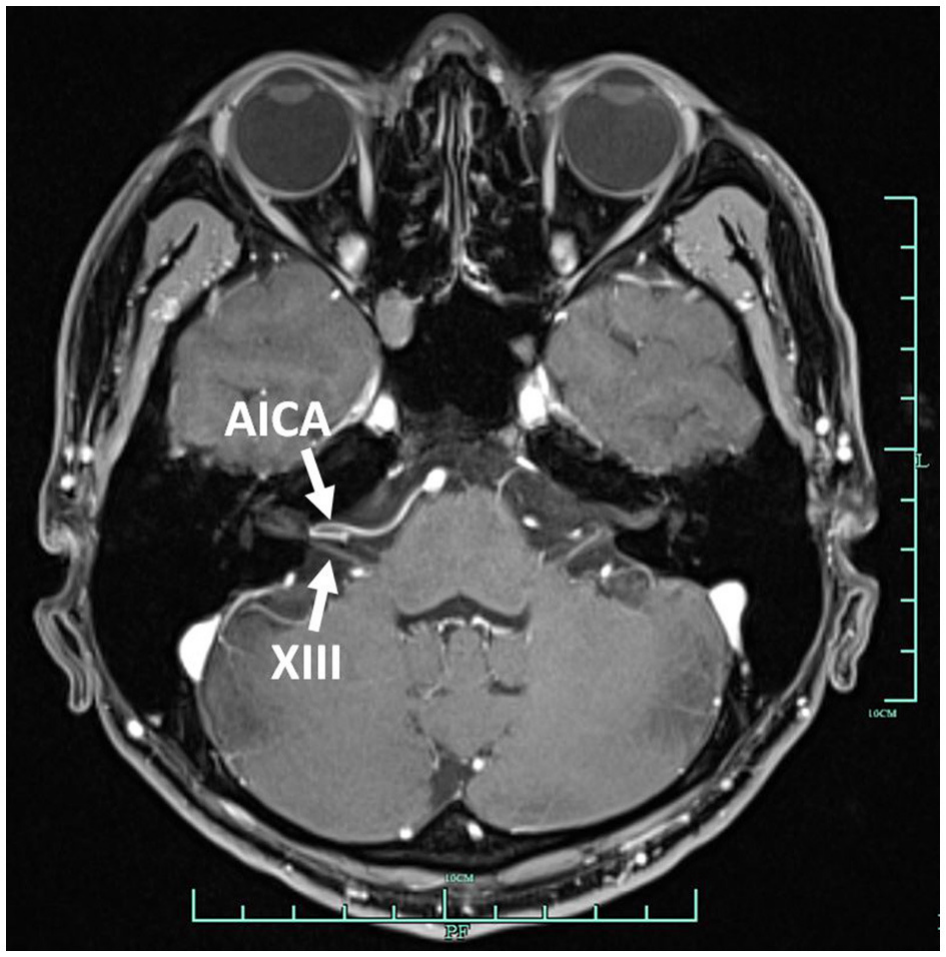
Contrast enhancement MRI of the internal auditory canal. The right AICA compressed the vestibulocochlear nerve. AICA, anterior inferior cerebellar artery; XIII, vestibulocochlear nerve.
